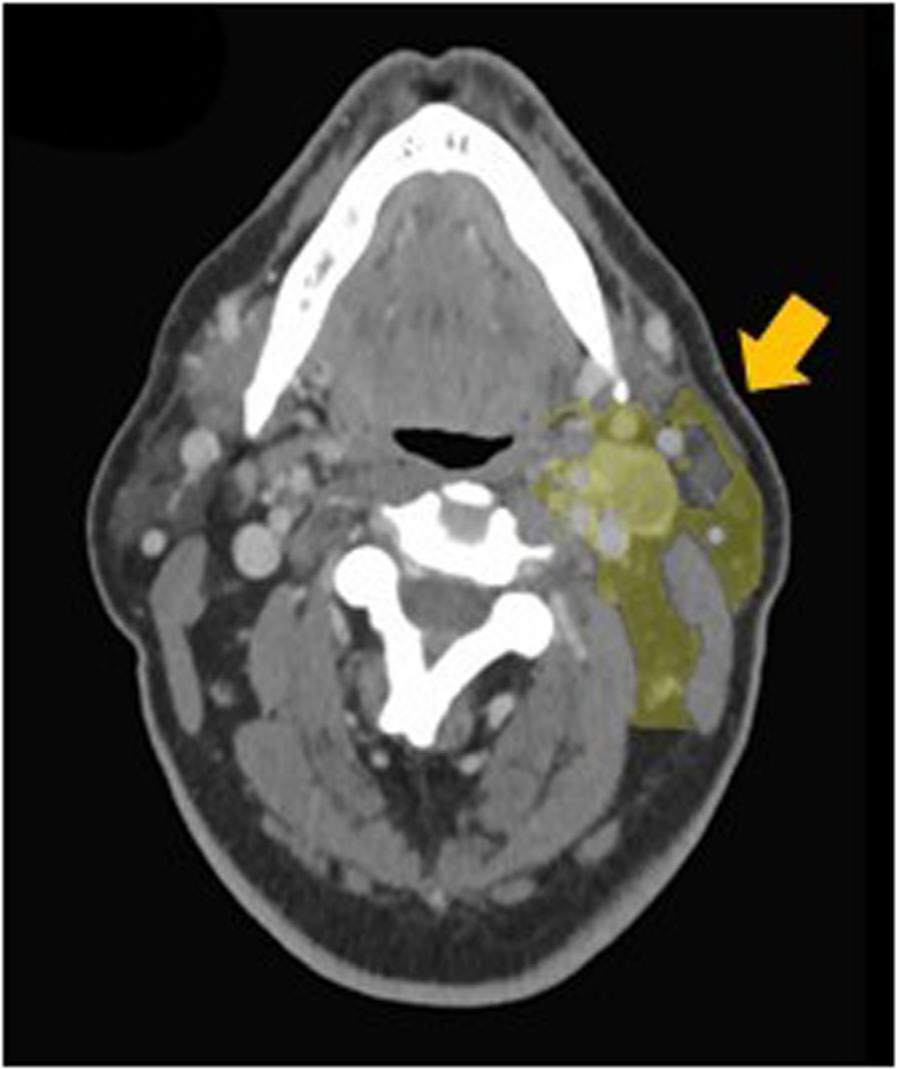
Segmented lymphatic tissue volumes on contrast-enhanced CT scans. Yellow highlighted regions correspond to the volume defined as level II-IV lymphatic tissue by Mimics following the processing steps described in Fig. 2
